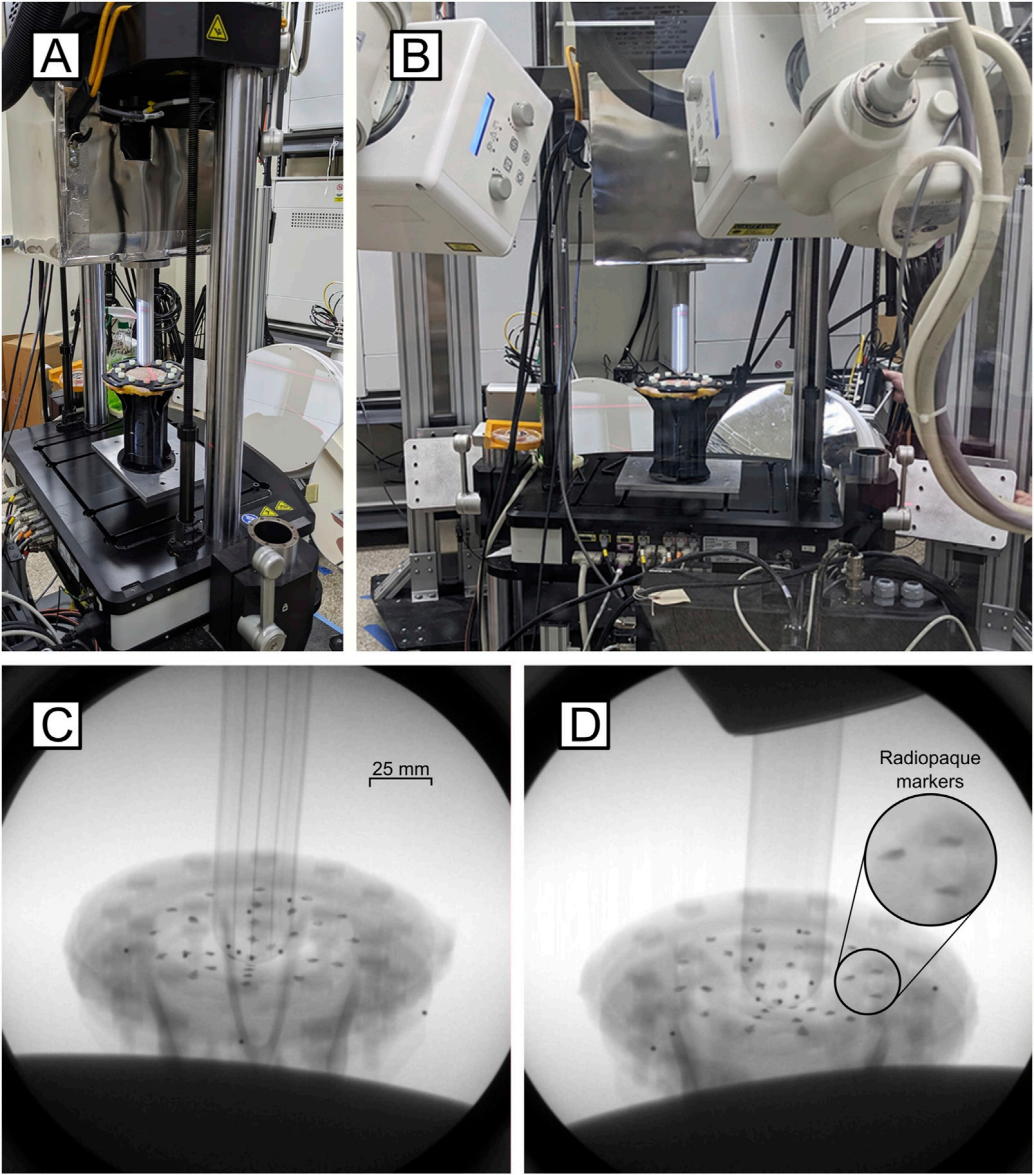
Validation apparatus setup. (A) The mechanical testing system interfaces with the DSX System. (B) Full view of the testing setup showing testing inclination. (C) Inline and (D) offset x-ray images of the skin painted with radiopaque markers during extrusion testing.
